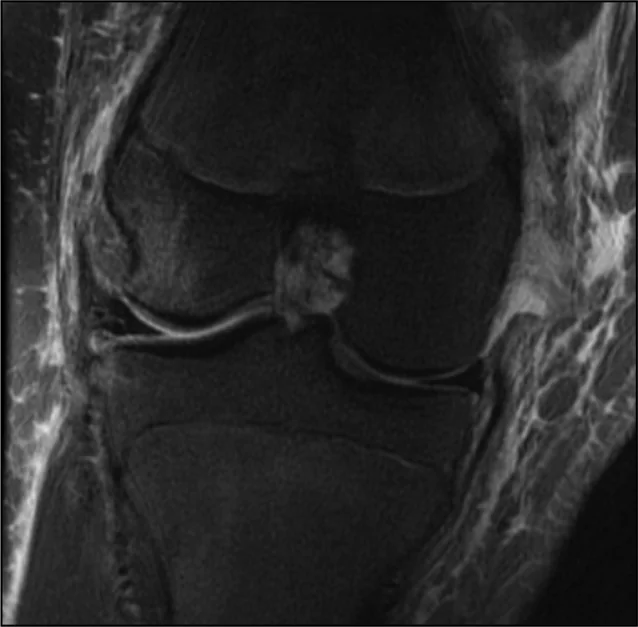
Coronal MRI demonstrating femoral avulsion of MCL and signal changes suggestive of a radial lateral meniscus tear.
